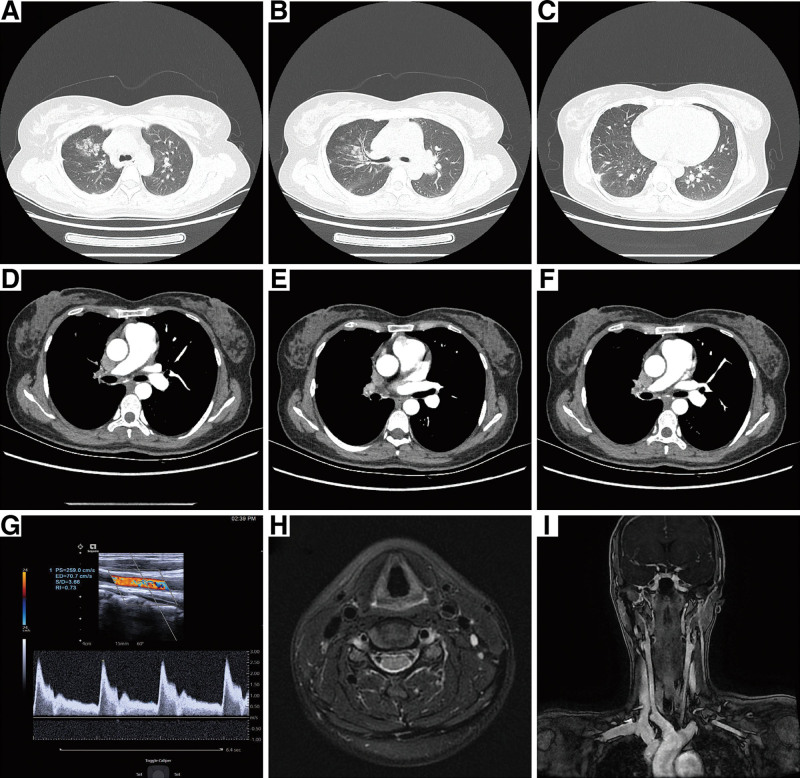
Pulmonary CT and aortic CTA. (A,B) Pulmonary CT showed ground glass exudation scattered in the right upper lung and considered the bleeding focus. (C) Subpleural cuneiform shadow. (D) Pulmonary enhanced CT showed the complete occlusion of the right pulmonary trunk and PAH. (E,F) The right bronchial artery was twisted around the right bronchus, forming a collateral circulation. (G) Color Doppler ultrasound of cervical vessels showed the diffuse and centripetal thickening of the wall and stenosis of the lumen from the beginning to the middle and distal segment of the left common carotid artery. (H,I) MRA showed the thickening of the left common carotid artery wall and a narrow lumen, and considered TA (inactive phase). CT = computed tomography, CTA = computed tomography angiography, MRA = magnetic resonance enhanced carotid angiography, PAH = pulmonary arterial hypertension.
